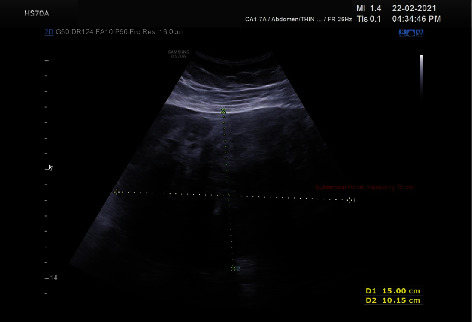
Ultrasound of the lower abdomen showing fibroid measuring about 15 cm.
